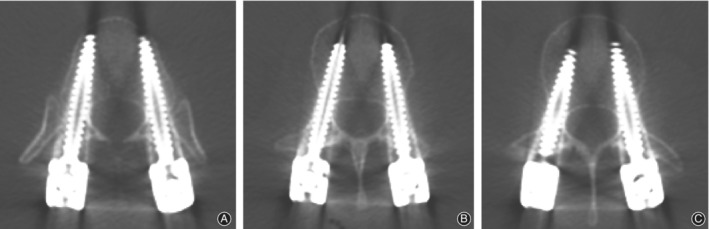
CT images of the above patient. (A) Postoperative CT axial images of T_12_. (B) Postoperative CT axial images of L_1_. (C) Postoperative CT axial images of L_2_.
